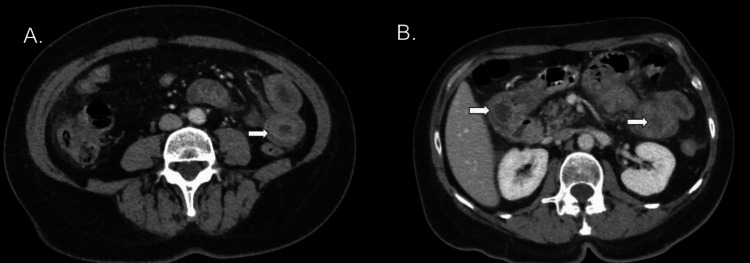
Axial contrast-enhanced CT abdomen and pelvis demonstrating findings consistent with ACEI-induced small bowel angioedema (A) August 2020; (B) December 2022. White arrows highlight the characteristic "target sign" and segmental bowel wall thickening. These images are original diagnostic CT scans from the patient described in this report; informed consent for publication was obtained.
